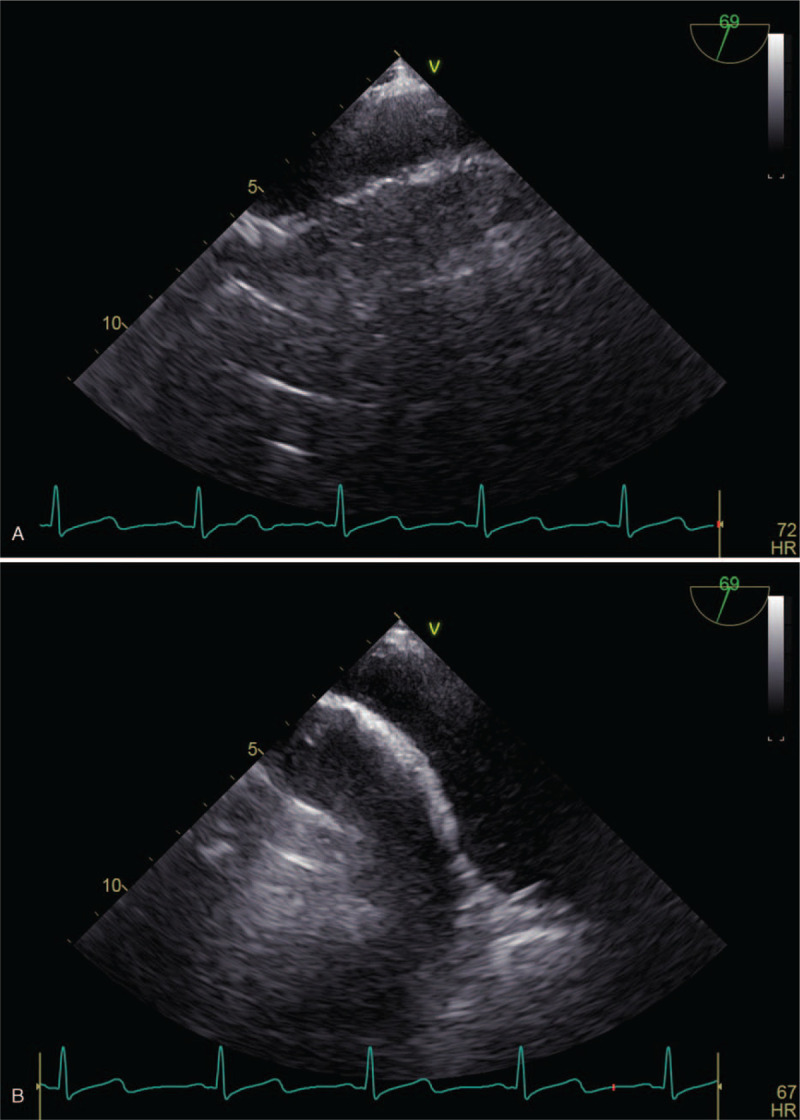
(A) TEE after the tumor thrombus was removed. (B) TEE after the tumor thrombus was removed. TEE = transesophageal echocardiography.
